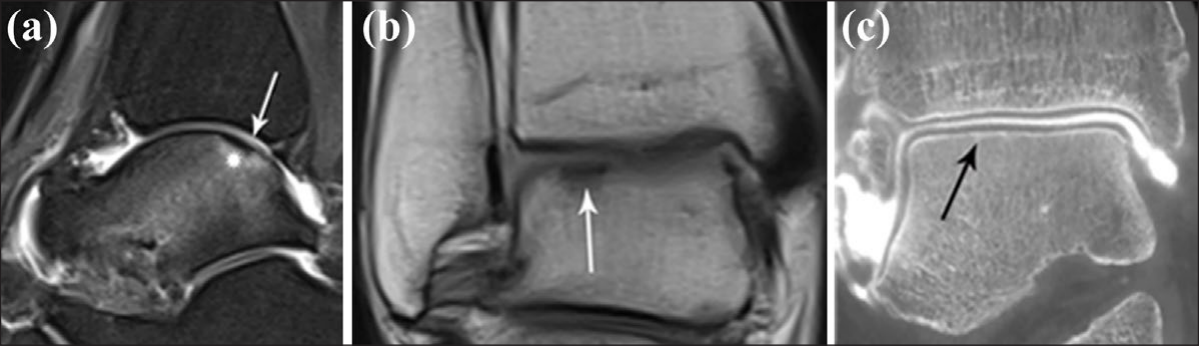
Alternative diagnosis on CBCT compared to MRI. Sagittal (a) fat suppressed T2-WI show a subchondral band-like area of low signal at the subchondral cortex (white arrow) with surrounding BME (white asterisk). Coronal proton density (b) shows focal hypointense thickening of the talar dome (arrow). Coronal reformatted CBCT-A (c) barely shows subtle subchondral sclerosis at the superolateral aspect of the talar dome and intact overlying cartilage. Based on the combination of MRI and CBCT findings the diagnosis of a subchondral insufficiency fracture (SIF) was made.
